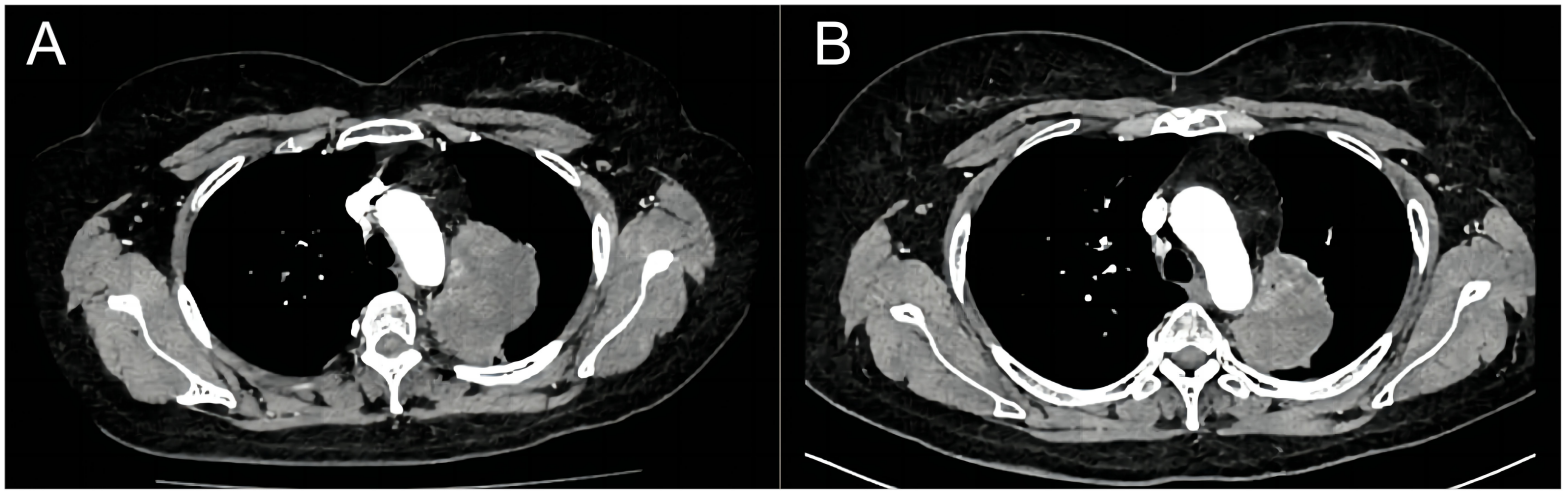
Computed tomography shows the comparison of lung lesions before (A) and after (B) the treatment of dabrafenib plus trametinib.
